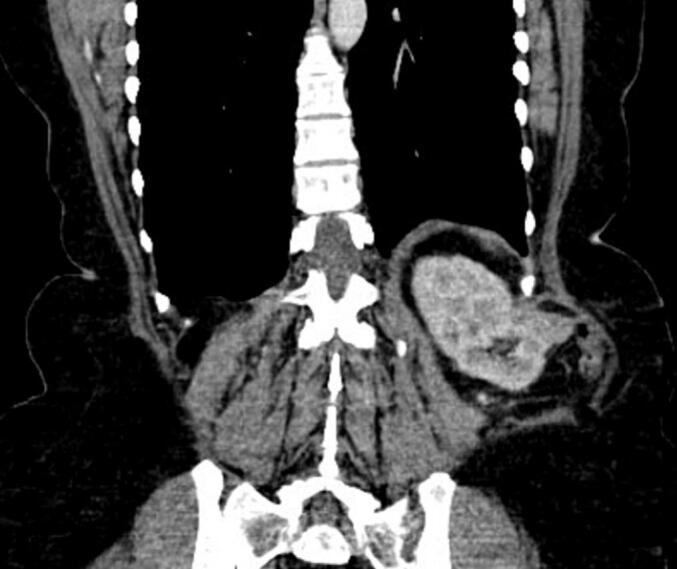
MRI findings revealed postoperative changes in the inferior pole of the kidney. In the lateral cortex of the left kidney, a 33 × 22 mm exophytic lesion was noted, which appeared isointense on T1-weighted imaging and hypointense on T2-weighted sequences, consistent with scar tissue. No restricted diffusion was observed. However, in the inferomedial surgical bed, a 5 × 9 mm T2 isointense focus with diffuse restriction and post-contrast enhancement raised suspicion for tumor recurrence. (Fig. 3., Fig. 4., Fig. 5., Fig. 6.).
